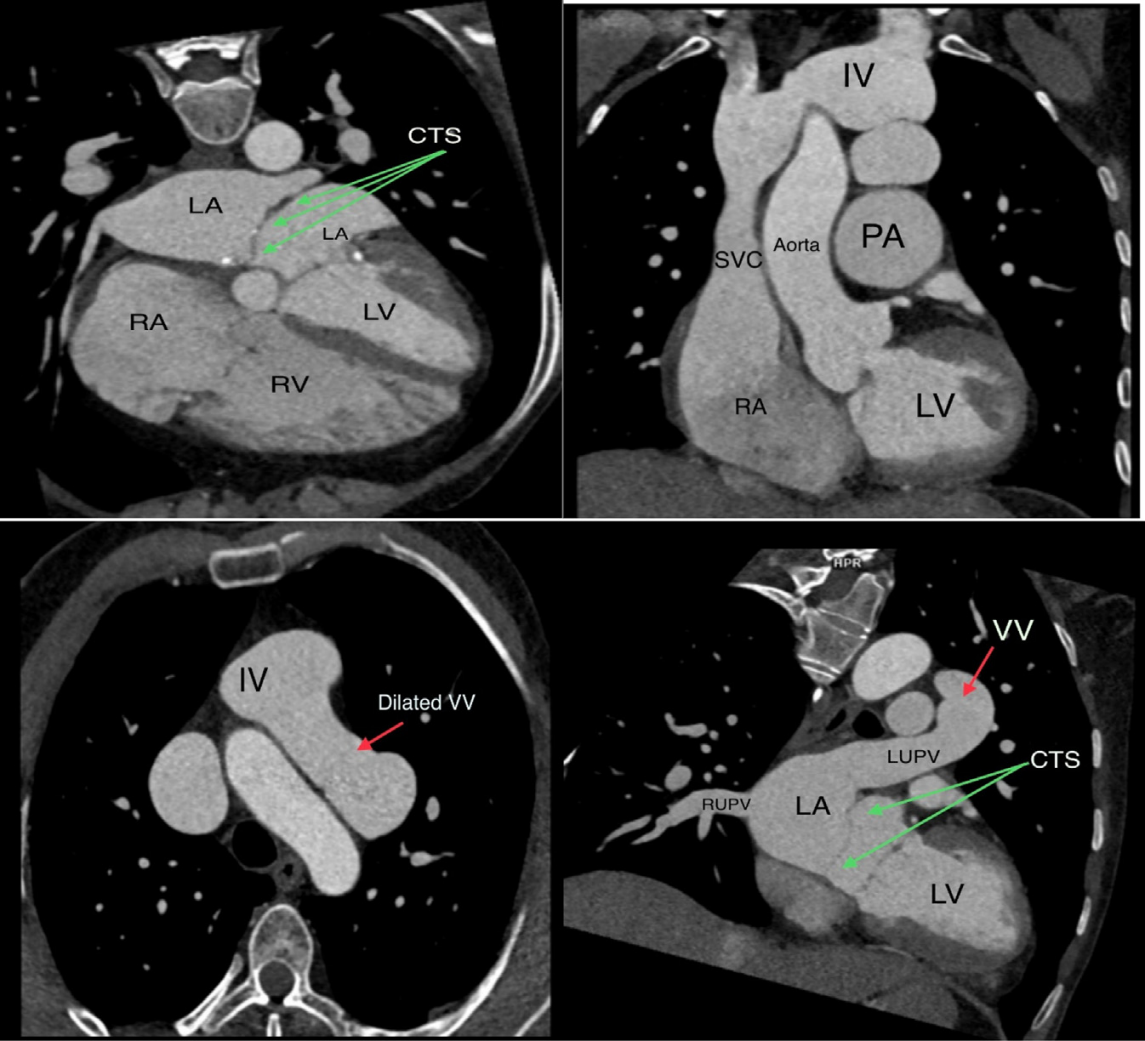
Contrast-Enhanced Multidetector Computed Tomography Contrast-enhanced multidetector computed tomography demonstrated dilated right side of the heart and dilated VV (red arrow) that terminated into the IV. Left atrium seen also divided into 2 cavities by CTS (green arrows). CTS = cor triatriatum sinister; LUPV = left upper pulmonary vein; RUPV = right upper pulmonary vein; SVC = superior vein cava; other abbreviations as in Figure 2.
